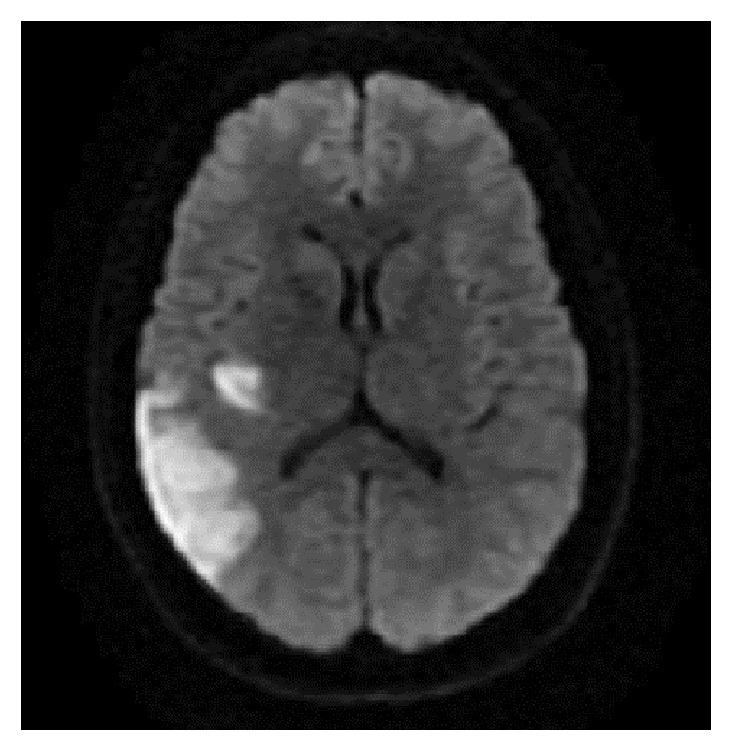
Axial images of diffusion-weighted MRI of the brain with contrast showing hyperintensity in the temporal and occipital lobes.
